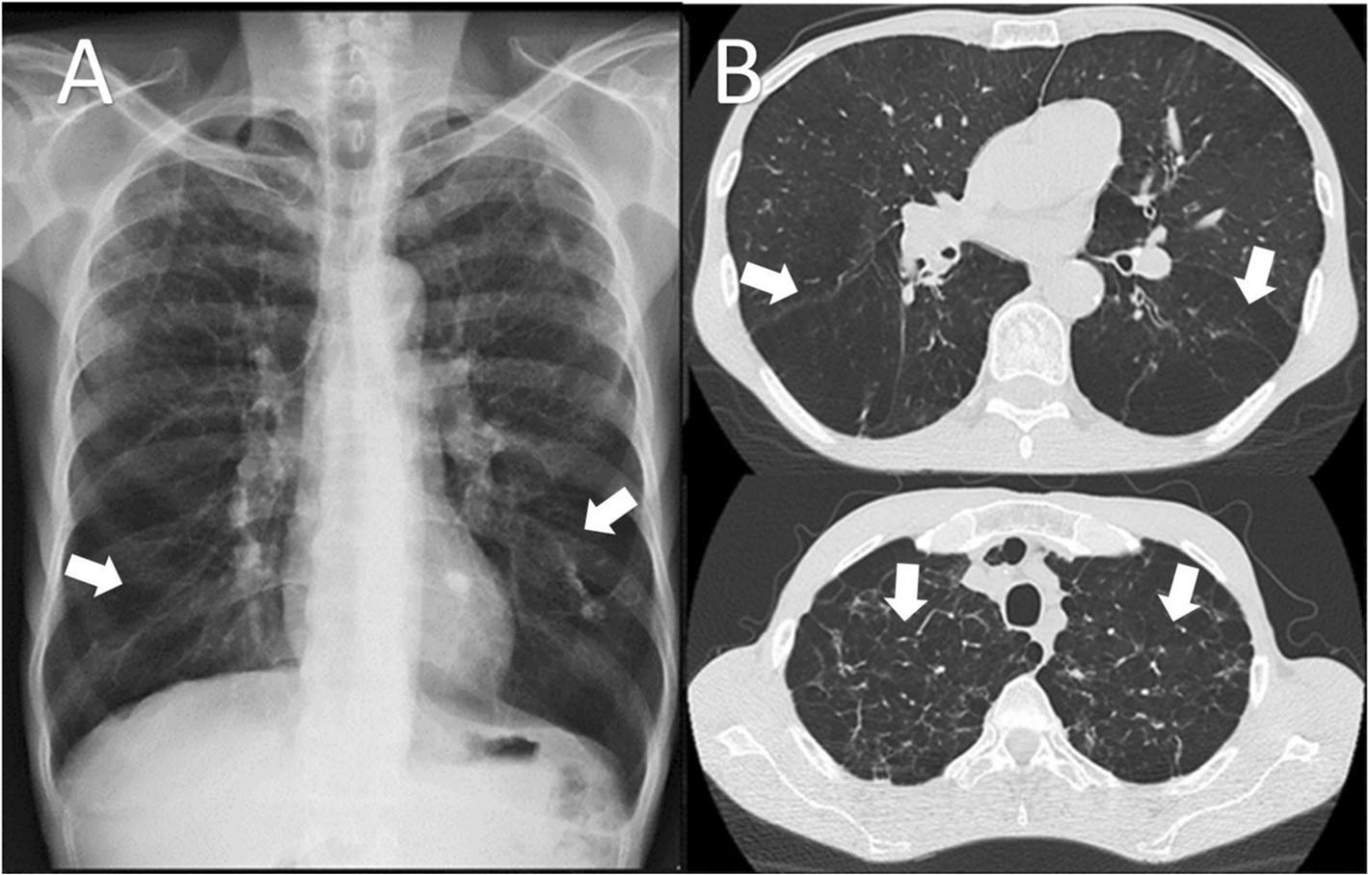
a Chest x-ray showing interstitial opacities and fibrotic tracts in the lung bases. b High Resolution Tomography of the chest. Lung window showing centrilobular and paraseptal emphysematous foci
